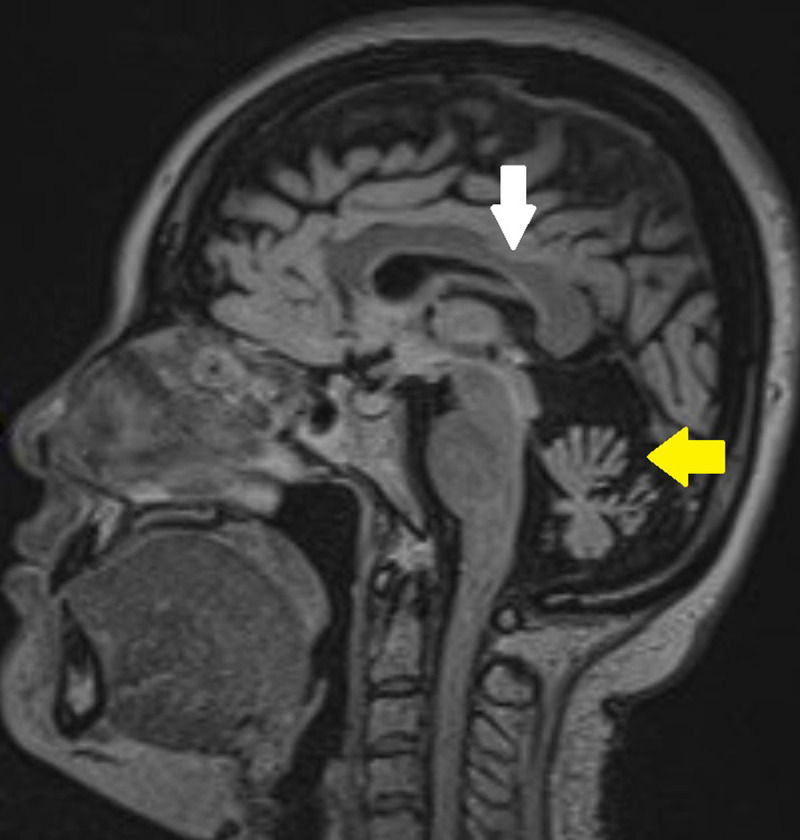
T2 FLAIR sagittal images showing atrophy of cerebellar hemispheres and the vermis (yellow arrows) with notching in the posterior part of body of corpus callosum (white arrow).
